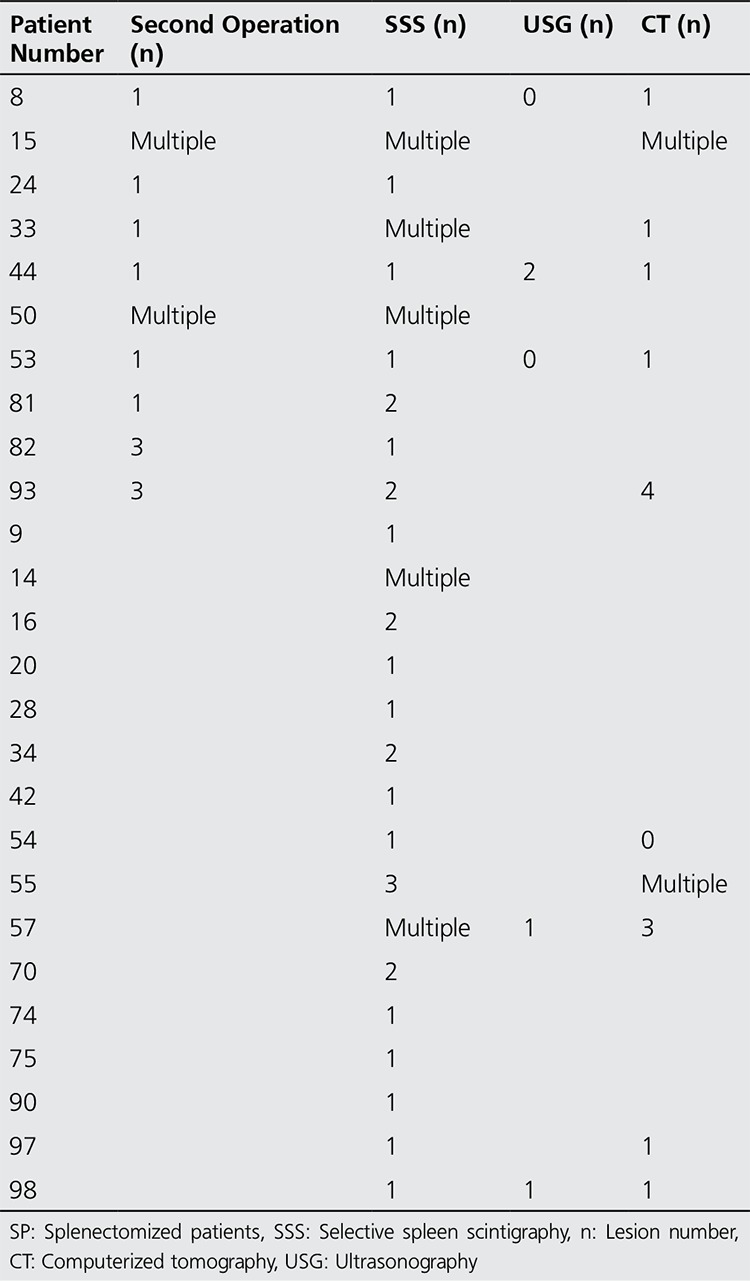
Comparison of selective spleen scintigraphy, ultrasonography, computerized tomography, and second operation results in splenectomized patients group
